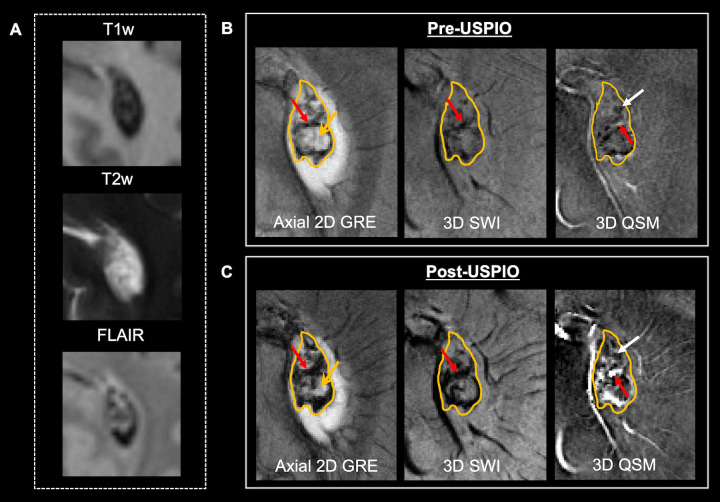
Contrast enhanced MRI with USPIO. (A) The ChP is not clearly depicted by conventional MR sequences, with obscure boundary between ChP and CSF. (B) Without USPIO, the boundary between CSF and ChP is clearly defined (yellow line) using 2D gradient echo on 7T, with hypointense vascular (red arrows), iso-intense stromal (yellow arrows), and hyperintense CSF components. The venous vessels are dark on SWI whereas bright on QSM. (C) After the administration of USPIO, both venous and arterial vessels are highlighted. In QSM data, the susceptibility value increases in vessels after contrast administration, whereas the calcium (white arrows) remains dark due to the diamagnetic property.
